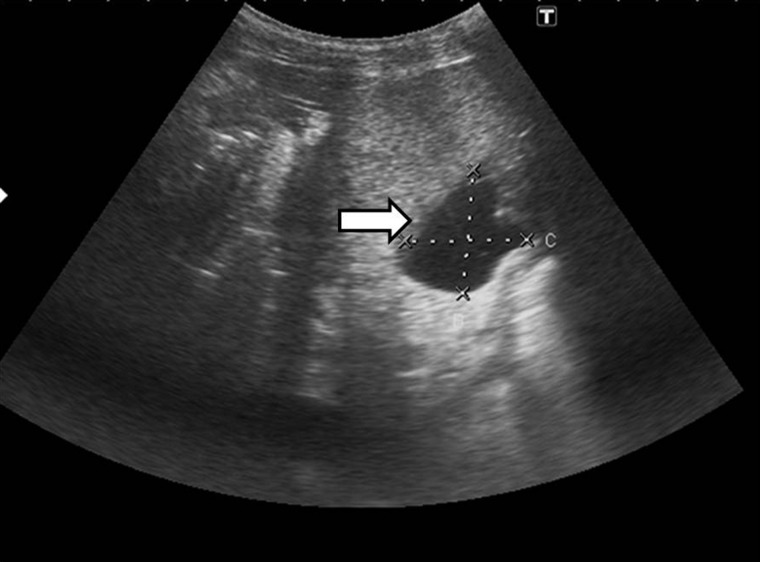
Seroma. Ultrasound image showing collection in the perihepatic location (arrow).
